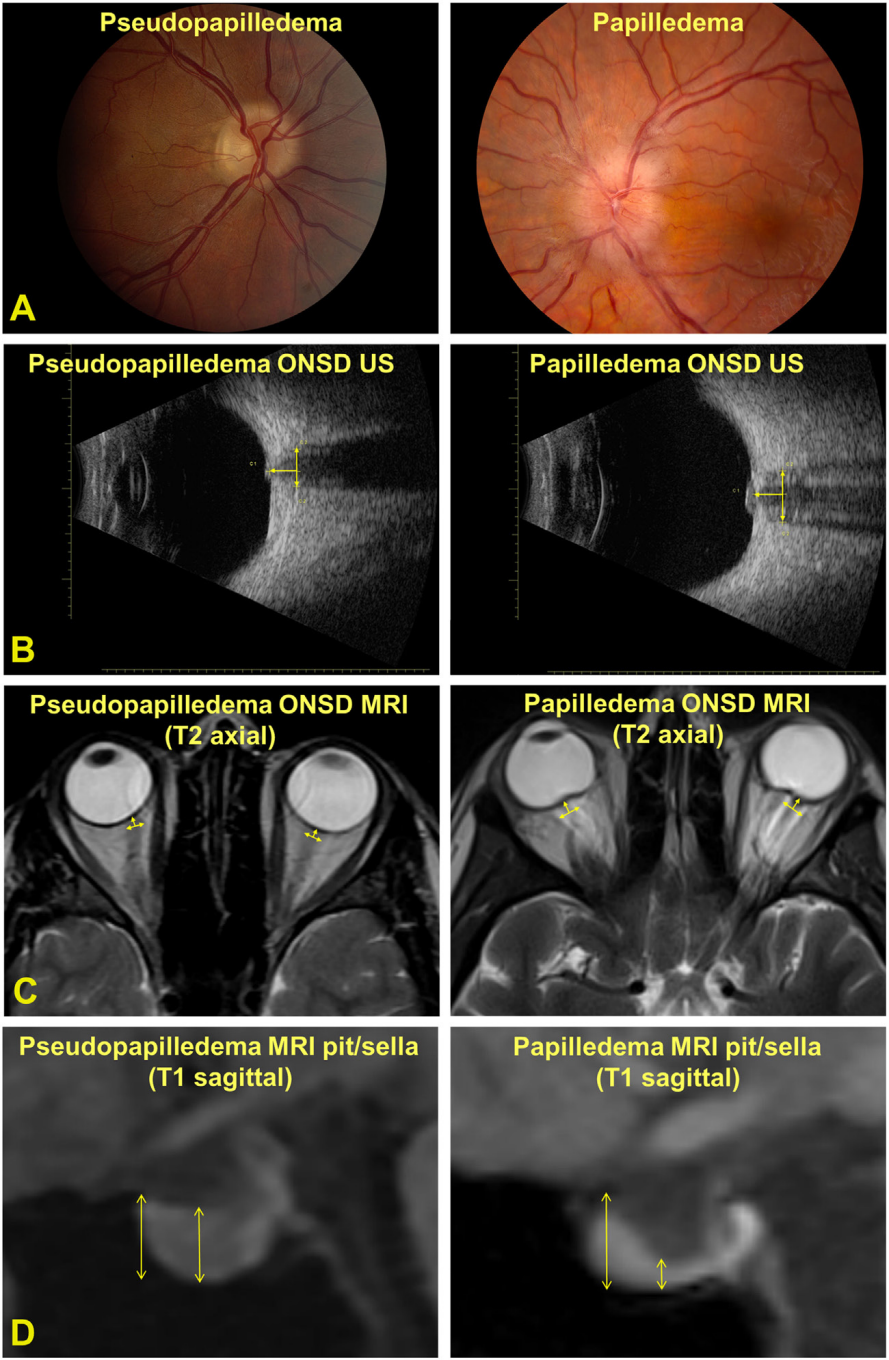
(A) Photographs of an optic nerve with pseudopapilledema (left panel) and papilledema (right panel). (B) ultrasonography- (US) measured optic nerve sheath diameter (ONSD) measured 3 mm posterior to the inner scleral surface. (C) Magnetic resonance imaging (MRI)-measured ONSD 3 mm posterior to the inner scleral surface. (D) MRI-measured pituitary (pit) and sella turcica (sella) measurements.
